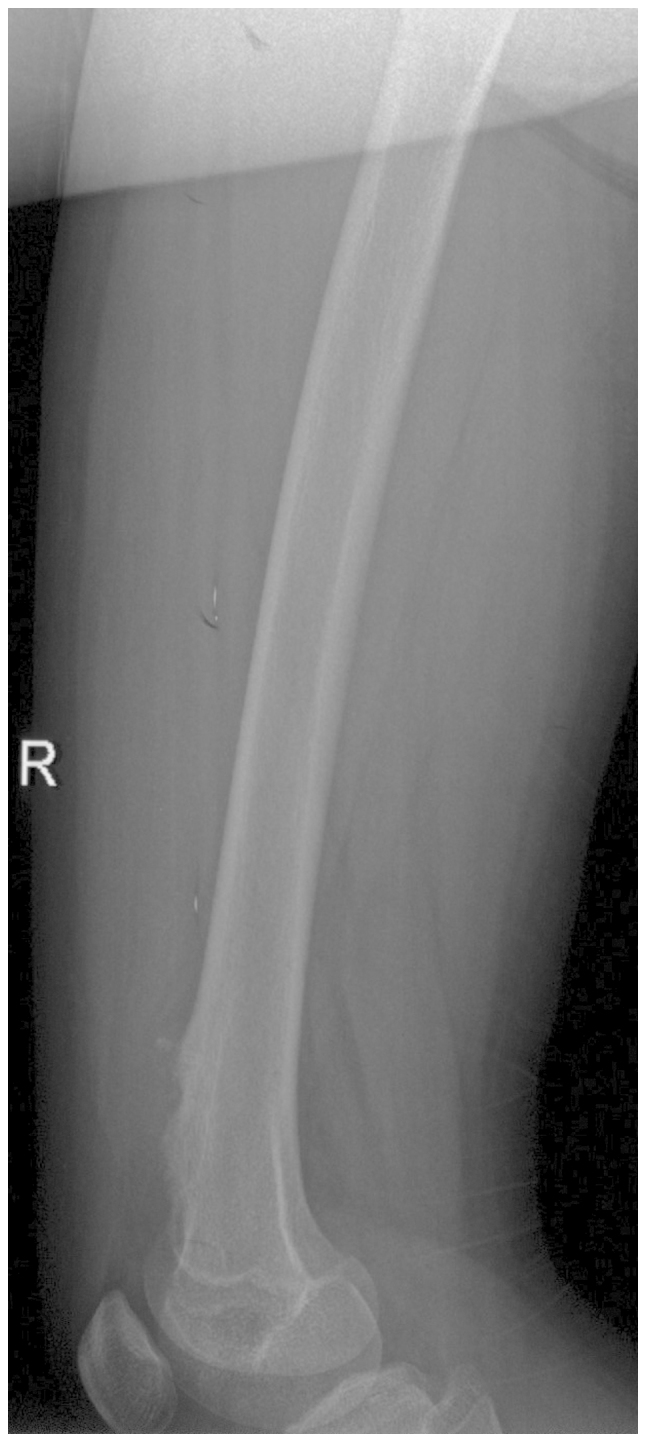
Radiography showed a large radiolucent shadow with stippled calcification.
